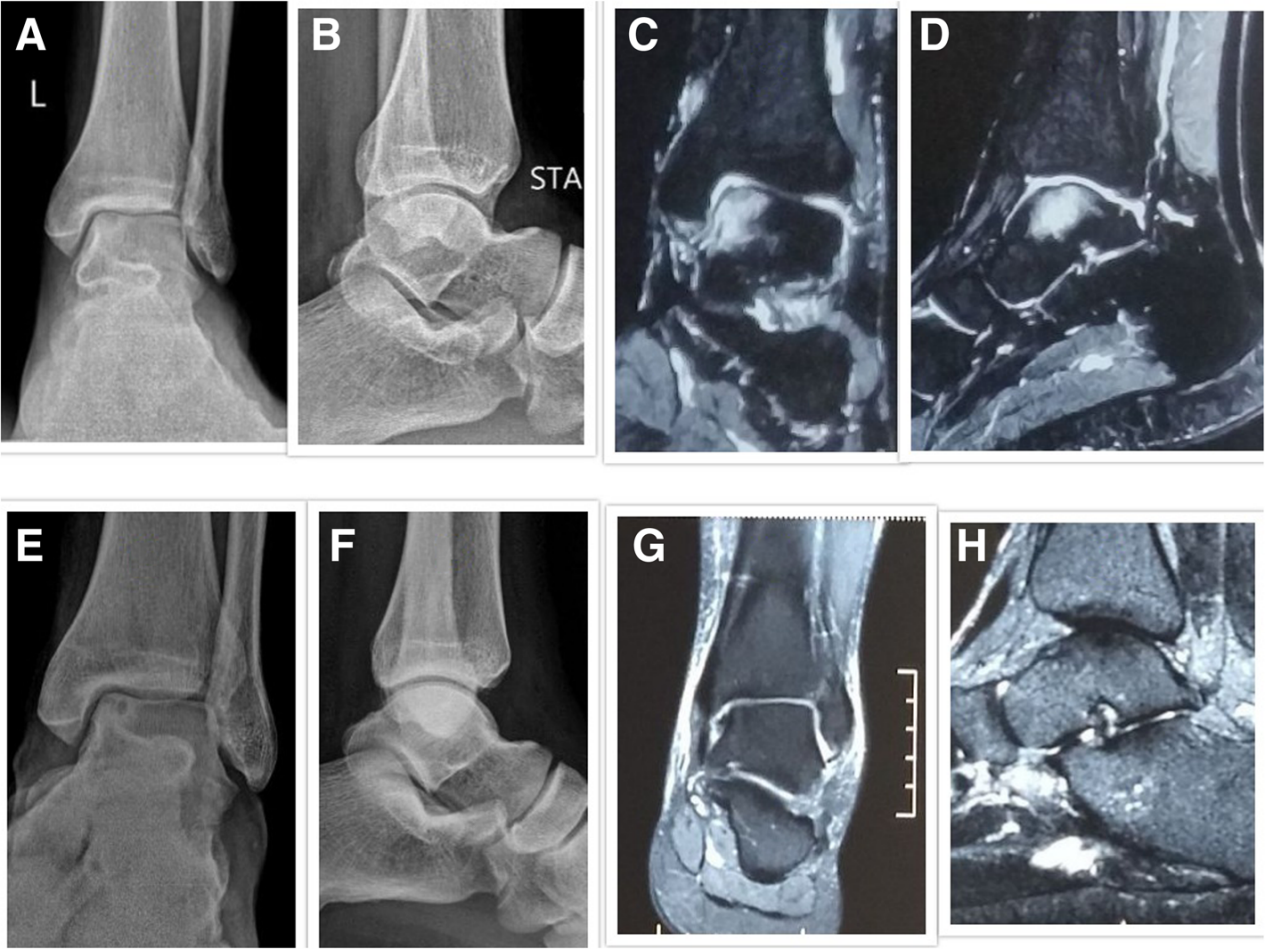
a, b Pre-treatment anteroposterior and lateral radiographs of a 50-year-old male diagnosed with modified Ficat and Arlet stage II avascular necrosis of the talus bone. c, d Pre-treatment MRI Coronal and Sagittal images showing BME. e, f Post-treatment anteroposterior and lateral radiographs at 50 months follow-up showing no radiological collapse/progression with stabilization of the AVN in stage II. g, h Post-treatment MRI coronal and sagittal images showing complete resolution of BME
